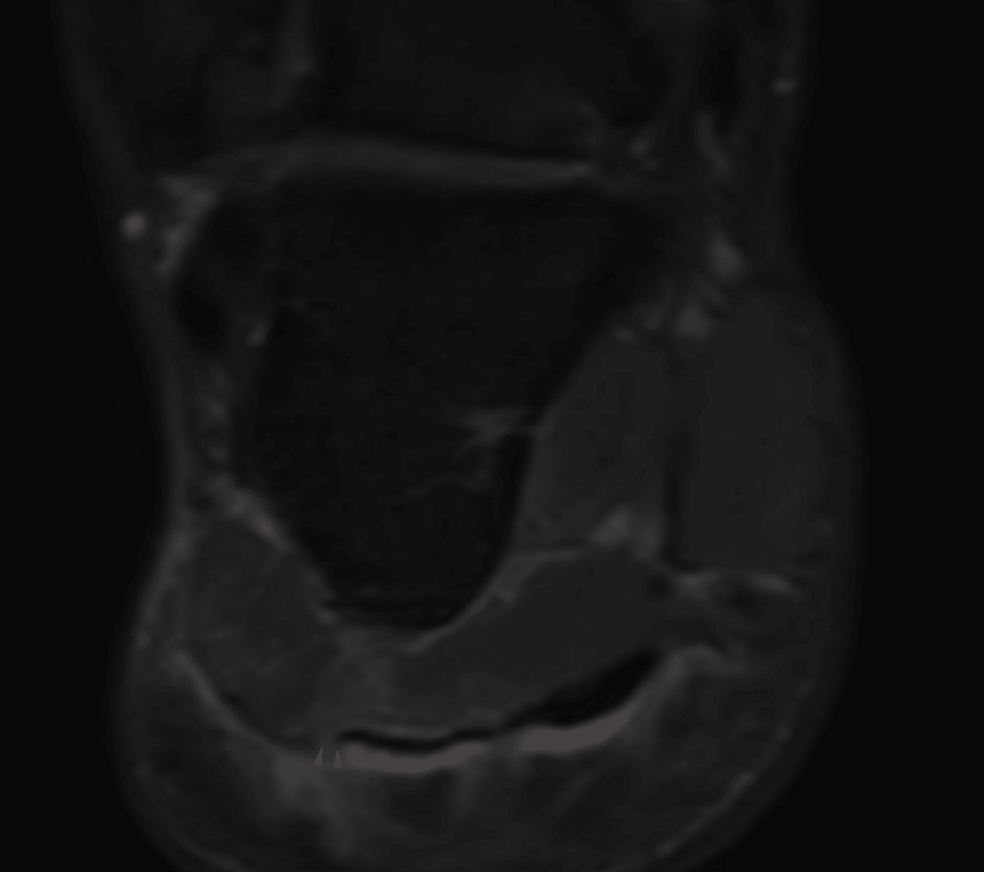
Coronal cut of proton-density MRI. Tear of the plantar fascia (red arrows).
